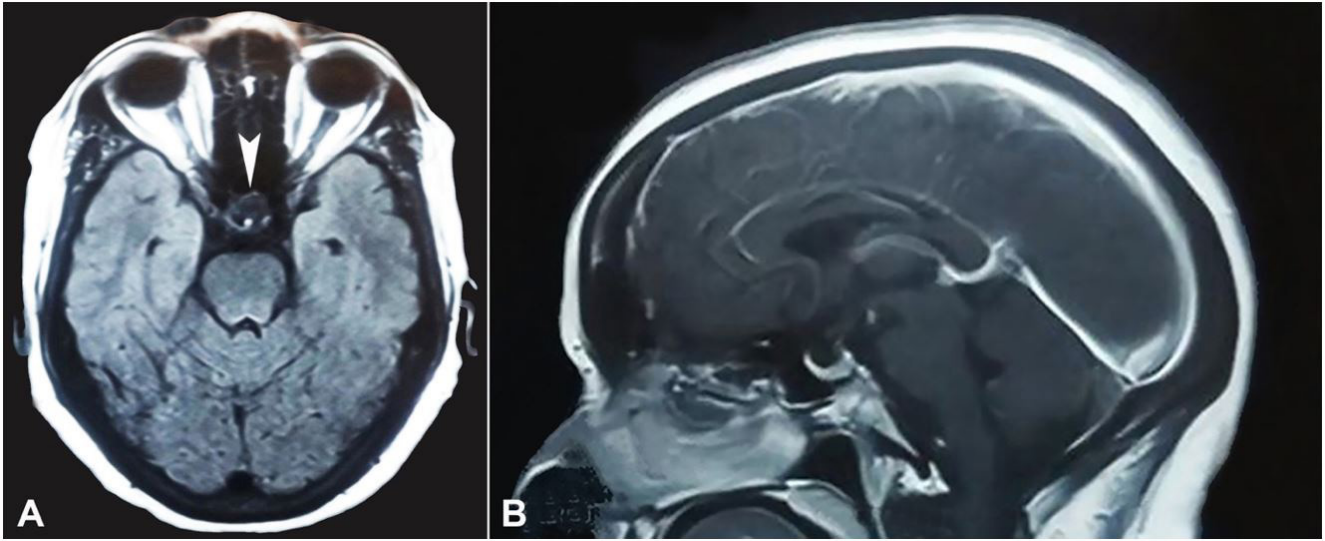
Brain MRI. A – T1W axial, and B – Sagittal Plane - Strongly enhancing a mass lesion measuring 1.7 x 1.4 cm arising from supra sellar region causing compression over optic chiasma without intrasellar or parasellar extension (arrowhead).
